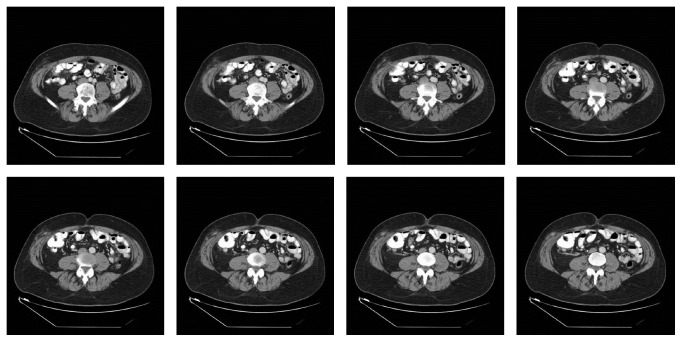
Axial images from a preoperative computed tomography scan demonstrating a right lower quadrant hernia containing fat and the appendix.
